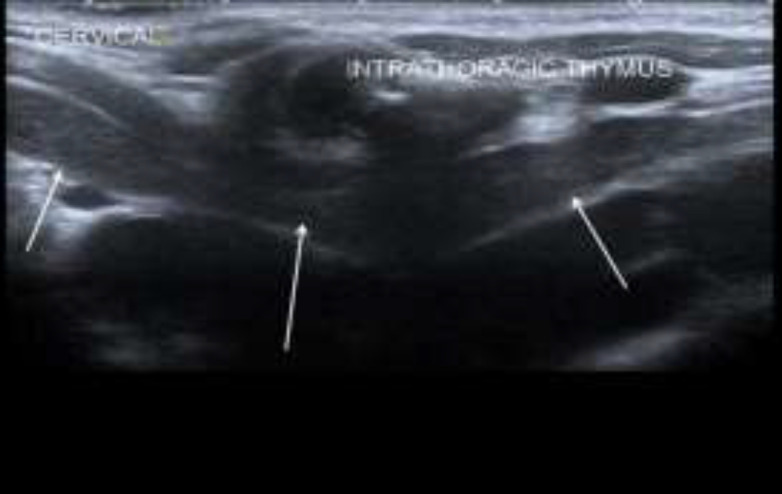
Ultrasound scan taken in sagittal view at left parasternal region shows thymus in the thoracic inlet which is continuous with the intrathoracic thymus (white arrows) when patient is at rest
